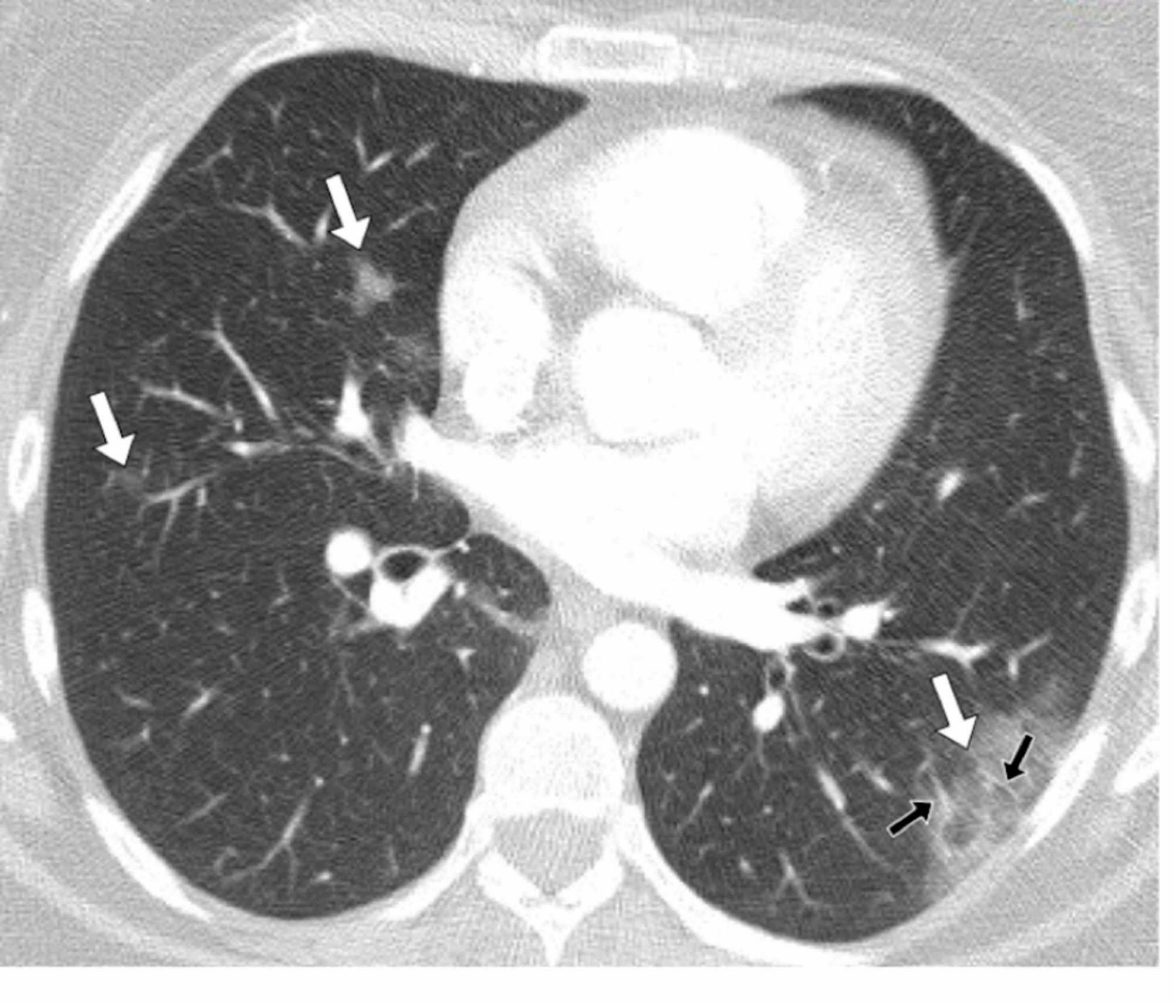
Patient 1 A 42-year-old female patient presented with a persistent cough and fever. Her D-dimer was 433 ng/mL and there was a clinical concern of a pulmonary embolus. A CT pulmonary angiogram (CTPA) scan was performed of the chest, which demonstrated changes consistent with COVID-19. A contrast-enhanced, axial CT image of the chest in lung windows showed bilateral patchy ground-glass opacification (white arrows). There was a large peripheral, posterior lesion in the left lower lobe in a distribution typical of COVID-19. There was also evidence of peripheral vascular engorgement (black arrows).
